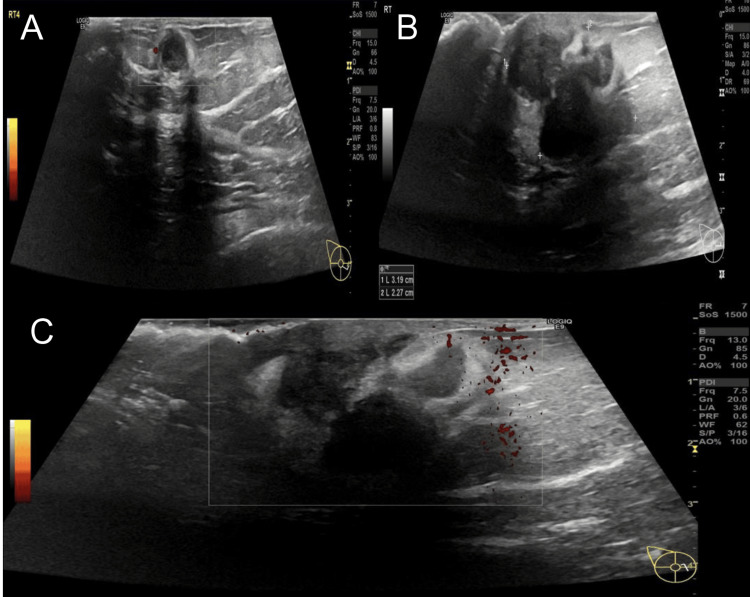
Right breast US of fibroglandular breast composition ACR type c A: Round-shaped, well-defined hypoechoic lesion seen in the right breast lower inner quadrant at 4 o’clock, with surrounding hyperechogenic rim, showing posterior acoustic enhancement. No internal vascularity was seen on the power Doppler. B and C: Amalgamated breast masses measuring about 32 mm x 24 mm (it was 11 mm x 10 mm) were seen encroaching the subareolar region along with posterior shadowing and overlying mild skin thickening and increased peripheral vascularity consistent with disease progression. No calcification, nipple or skin retraction, or lymphadenopathy were observed. ACR: American College of Radiology
